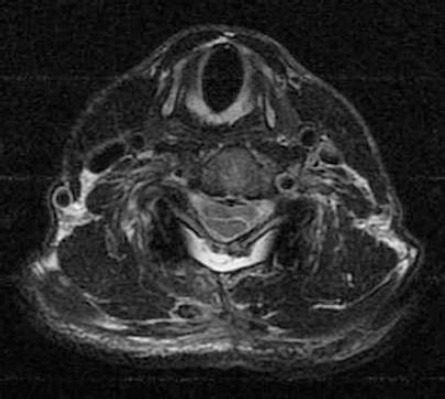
There is no arachnoid cyst in postoperative T2 MRI axial section*.
